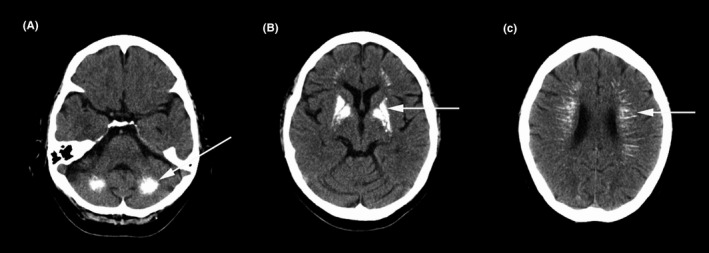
Head computed tomography scan showed symmetric calcified lesions in the dentate nucleus (A, arrow) and lenticular nucleus (B, arrow) bilaterally, as well as radiating high‐density lesions along the periventricular region (C, arrow)
